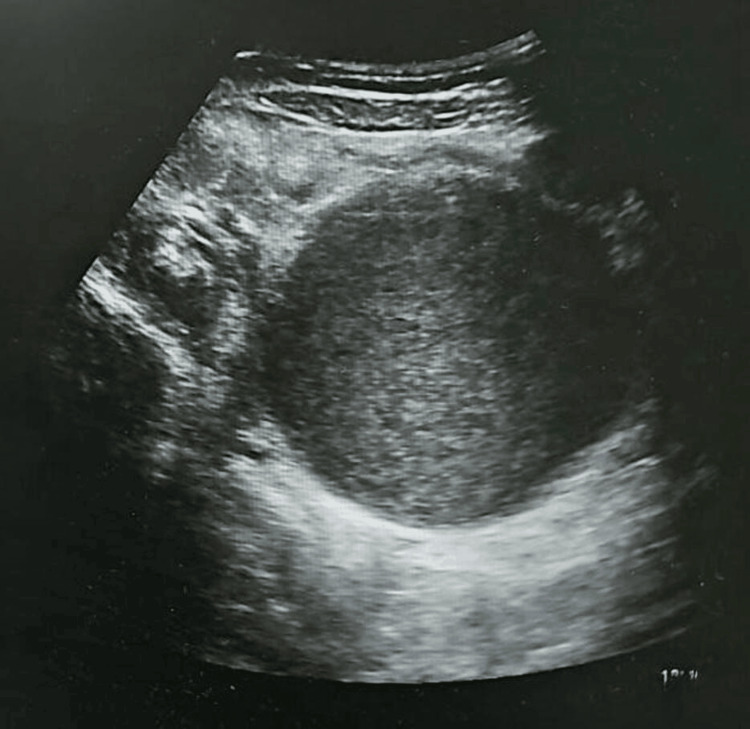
Pelvic point-of-care ultrasound demonstrating a distended, fluid-filled vagina and uterus consistent with hematocolpos.
